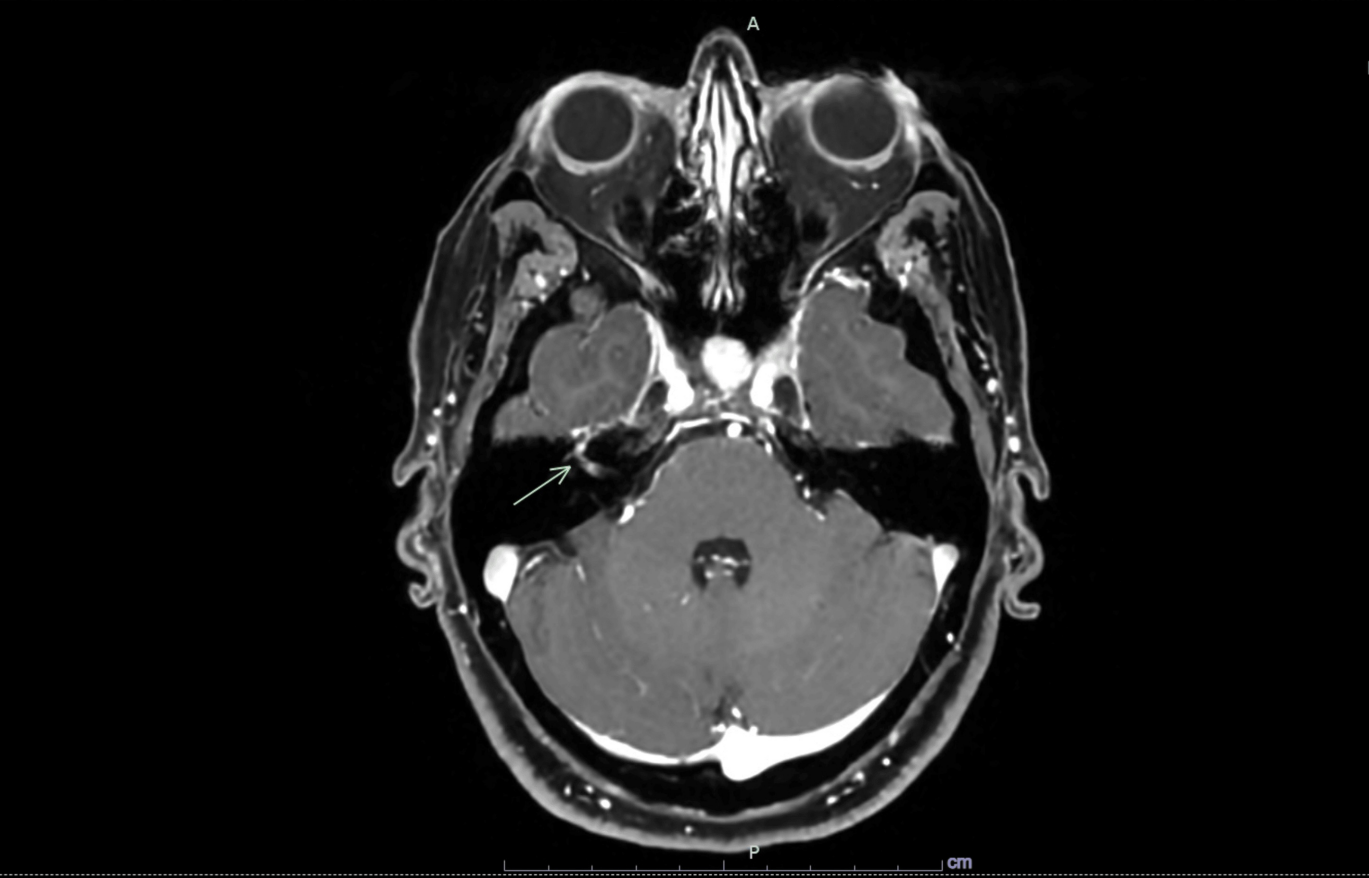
Axial MRI diffusion-weighted image showing increased enhancement of the distal labyrinthine segment of the right facial nerve distal, suggestive of facial nerve neuritis (green arrow). MRI, magnetic resonance imaging
